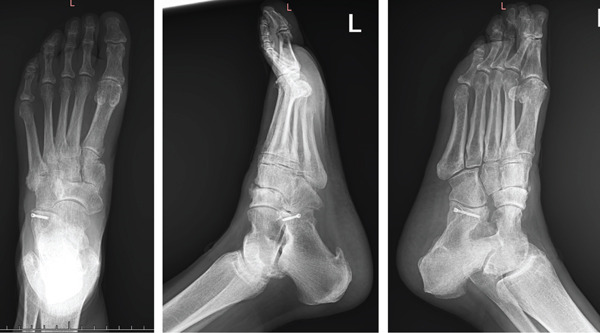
Anteroposterior, lateral, and oblique radiographs of left foot 15 months post‐op, note dorsal naviculocuneiform spurring.
